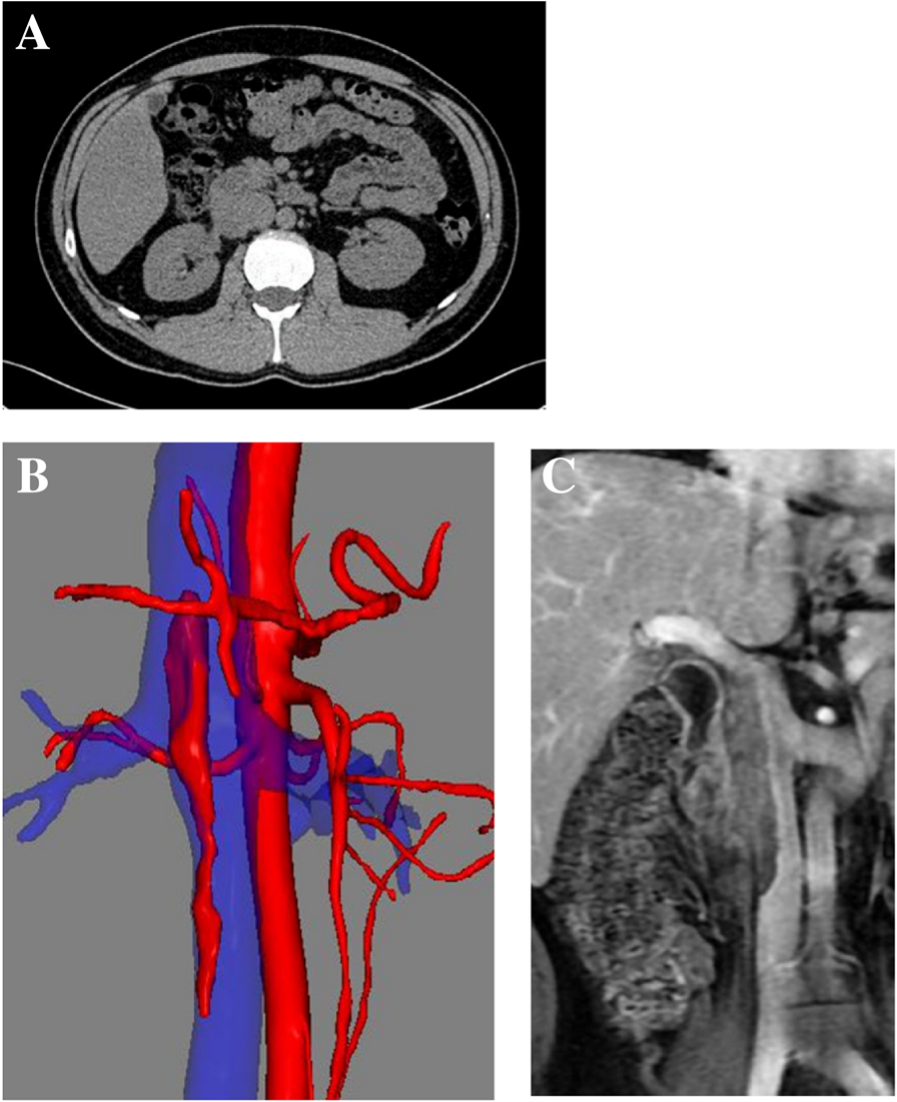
The lymph node enlargement on computed tomography image (a) and three-dimensional image reconstruction (b) of tumor thrombus in the vena cava and relevant magnetic resonance image (c)
